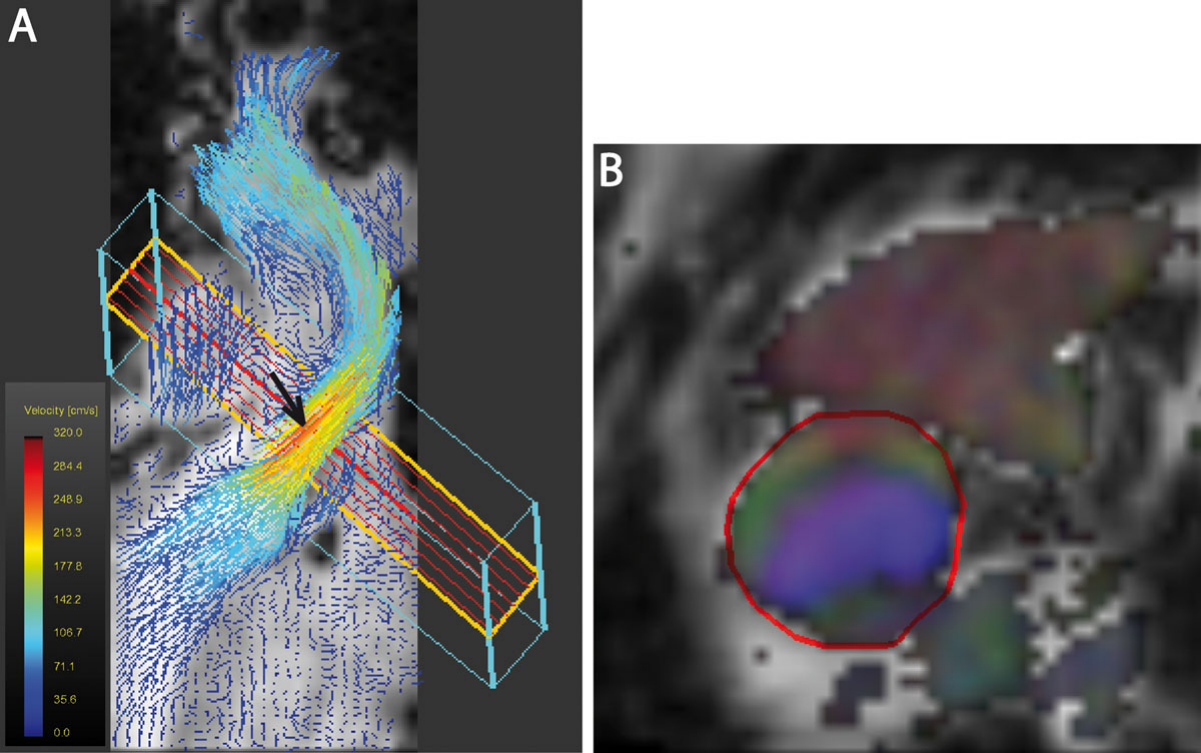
Assessment of peak velocity using flow visualization by 4D VEC MRI Figure: Velocity information in the left ventricular outflow tract of a patient with bicuspid aortic valve. A) Coronal axis of the left ventricular outflow tract, aortic valve and ascending aorta with in-plane visualization of velocity information. Dark red (black arrow) visualizes site of peak velocity. B) 4Dmax-targeted plane for blood flow quantification with colour coded flow direction.
